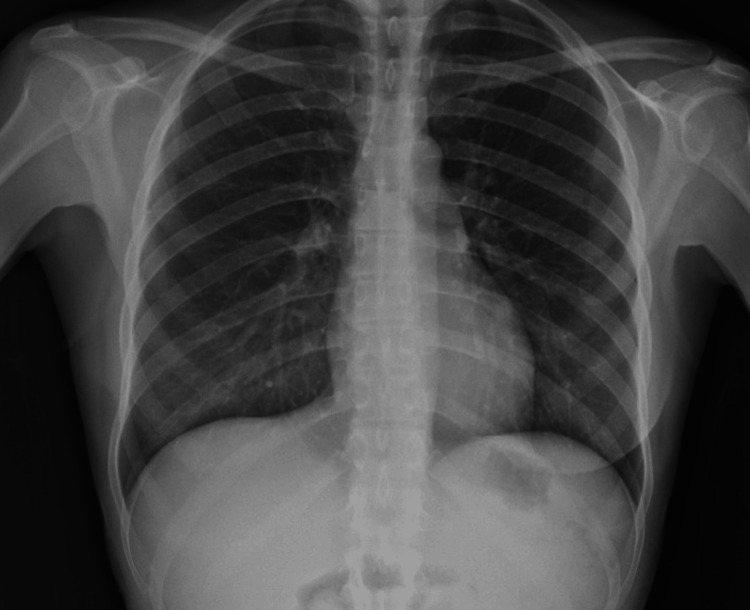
Chest X-ray at the discharge demonstrating the resolution of the hemothorax
